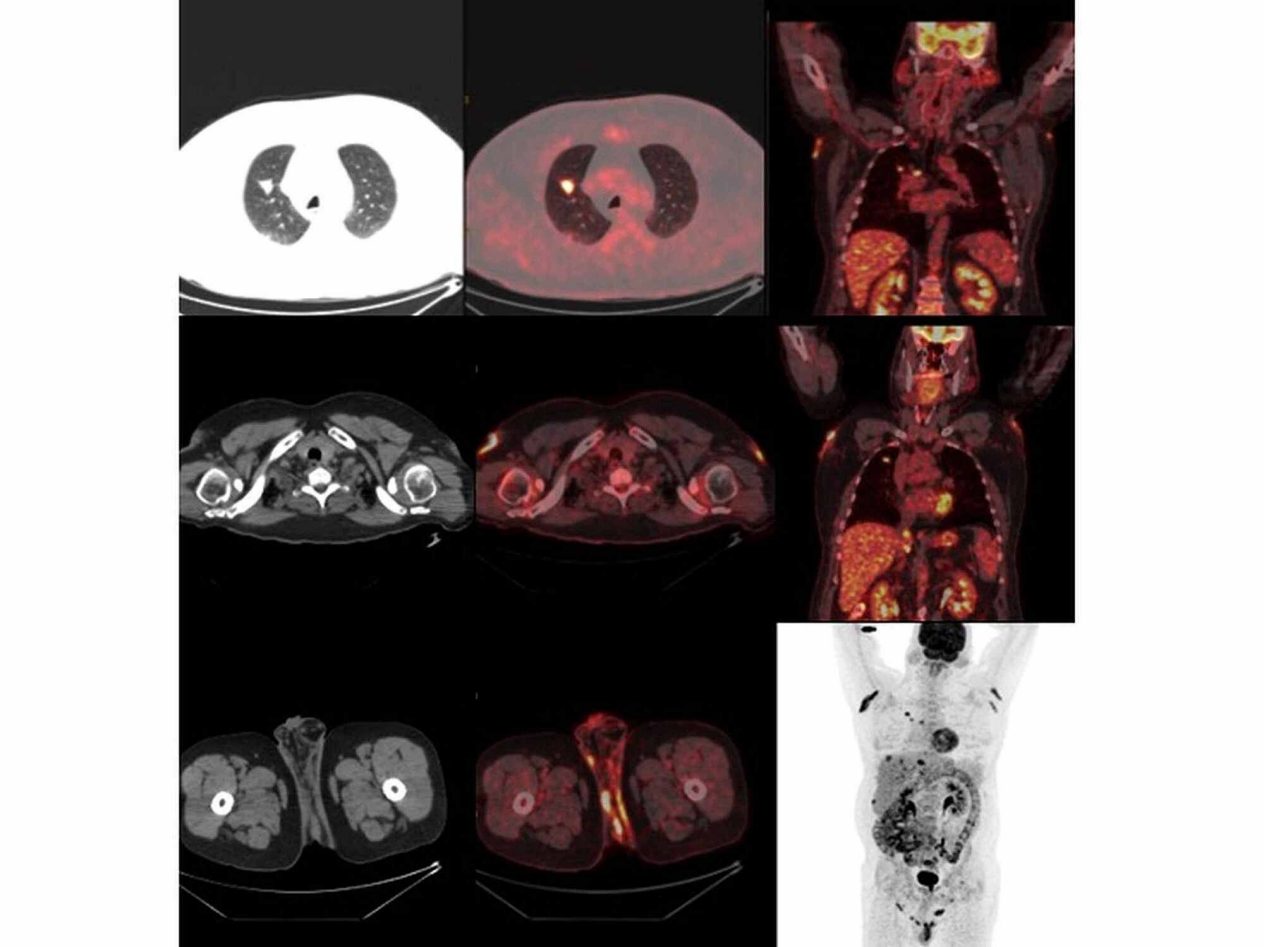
Primary tumor in lung and lesions in axillary and perianal region with increased FDG uptake
